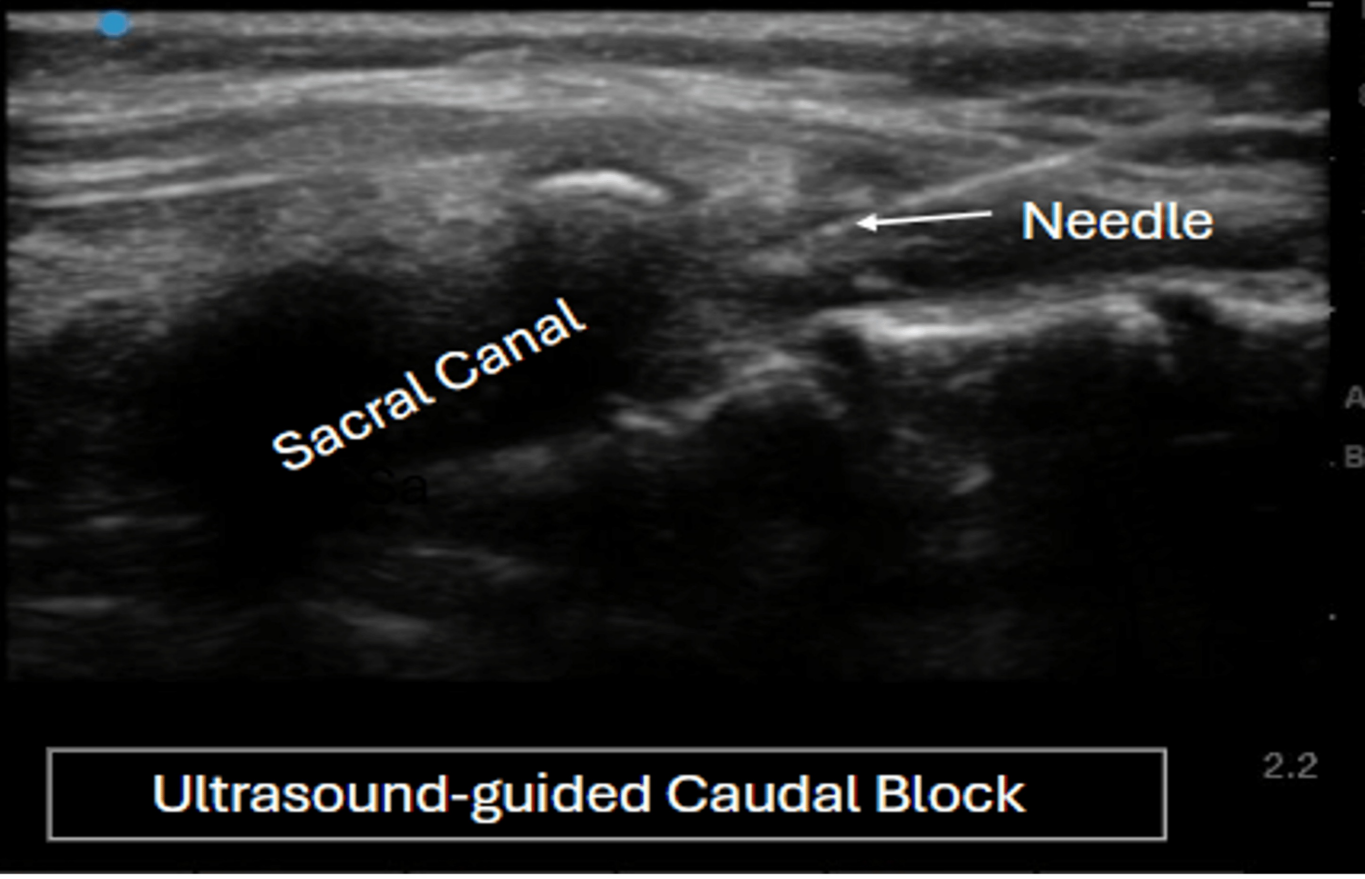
Ultrasound-guided caudal block for pain relief in Case 1
